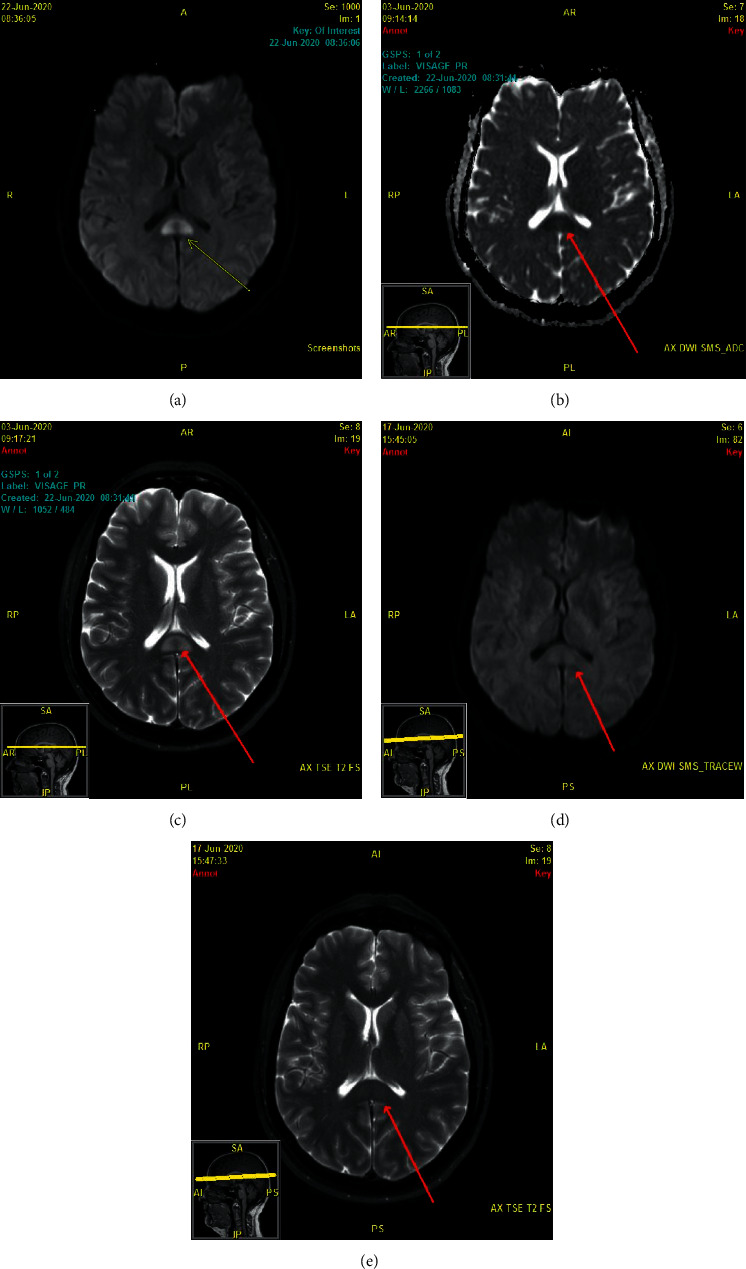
(a–c) MRI brain completed 16 days after lumbar puncture demonstrating an oval-shaped focus of abnormal unenhancing T2/FLAIR hyperintensity with restricted diffusion of the splenium of the corpus callosum (arrow). (a) Axial diffusion. (b) ADC mapping confirms true restricted diffusion. (c) Axial T2. (d, e) MRI brain completed 14 days after initial MRI with near-to-complete resolution of the corpus callosum lesion (arrow). (d) Axial diffusion. (e) Axial T2.
